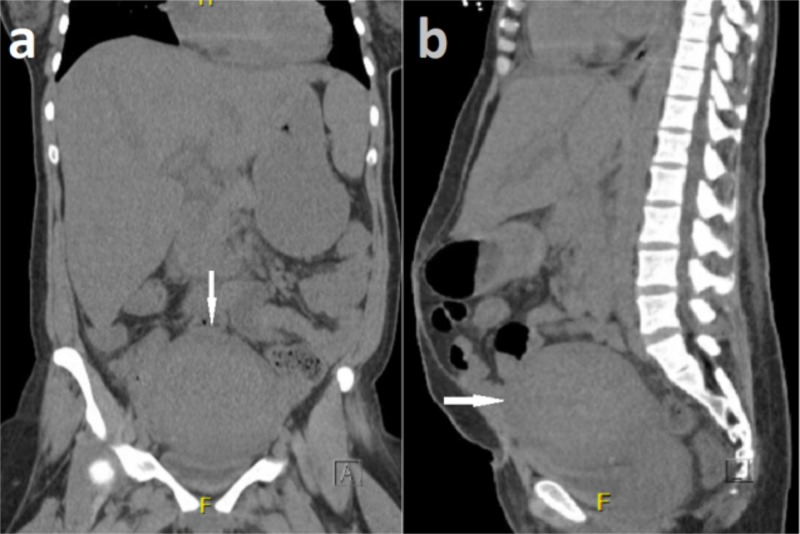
Coronal and sagittal plane computed tomography (a and b, respectively) of the abdomen and pelvis (with contrast) showing bulky uterus with no localized collection of fluid (white arrows)
